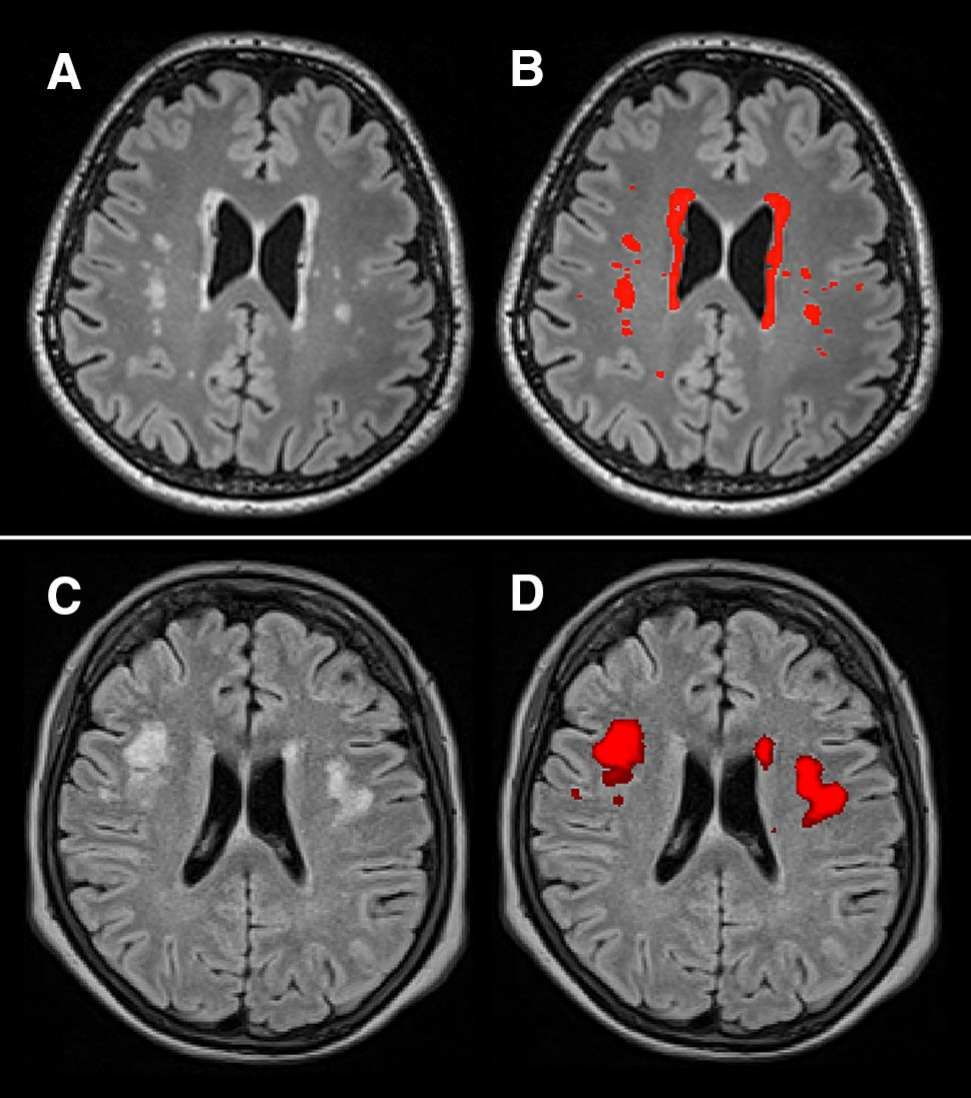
Example of manual WMH segmentation in the KORA sample (A,B) and automated WMH segmentation in the SHIP sample (C,D). (A) Axial view of the T2-weighted fluid attenuated inversion recovery data of a KORA participant with a WMH volume of 9600 mm^3^. (B) Manual segmentation of WMH in KORA. (C) Axial view of the T2-weighted fluid attenuated inversion recovery data of a SHIP participant with a WMH volume of 8600 mm^3^. (D) Automated multimodal segmentation of WMH in SHIP.
